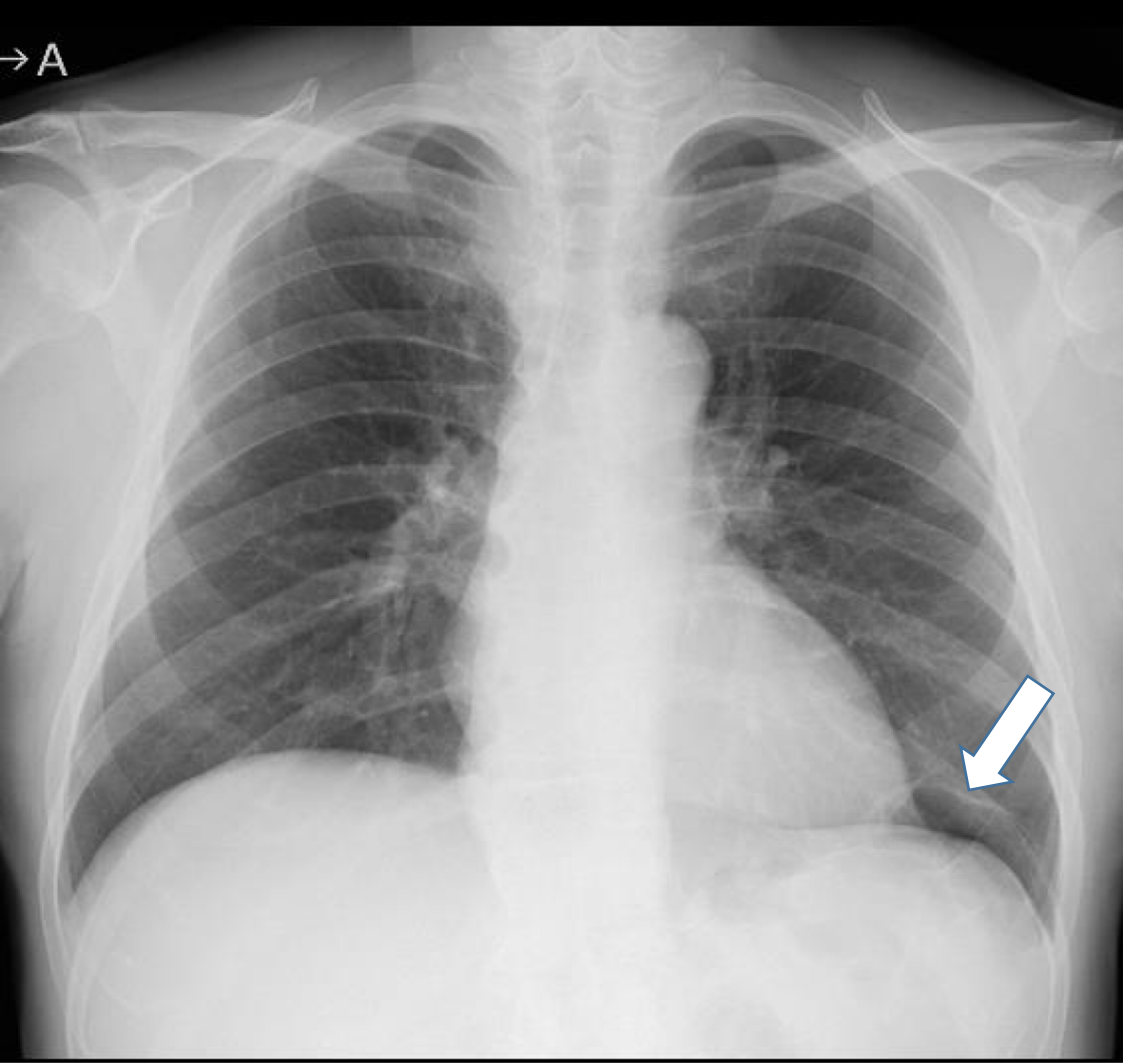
A chest radiograph showed a linear shadow in the left lower lung field (arrow)
